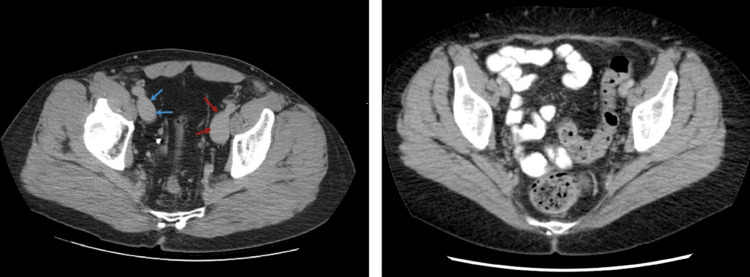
(Left) Dilated left (red arrows) and right (blue arrows) iliac vein (red arrows) in our patient; (Right) computed tomography at the same level in a normal person for comparison. (Right panel): Case courtesy of Andrew Dixon [5]. Permission was obtained from Radiopaedia.org.
